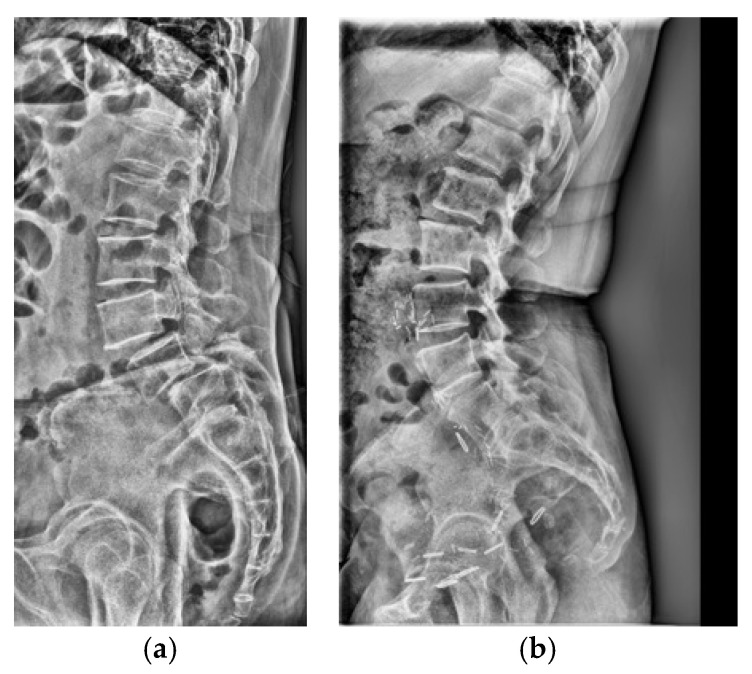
Representative misclassified cases. (a) Normal case misclassified as LSTV (false positive), showing subtle morphological features mimicking transitional anatomy. (b) LSTV case misclassified as normal (false negative), displaying transitional features similar to those in panel (a), which contributed to the classification difficulty on lateral radiographs.
